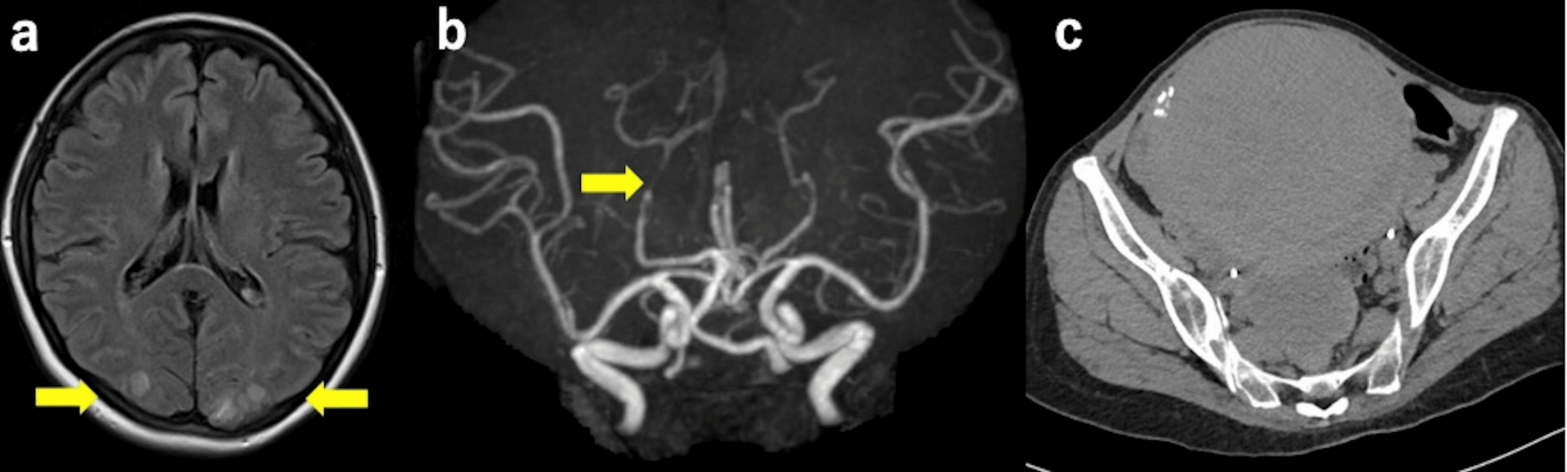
Axial FLAIR MRI (1.5 T; repetition time, 10,000 ms; echo time, 102 ms) on the day of PRES onset (a) shows bilateral hyperintensity in the occipital subcortical white matter (arrows) (a). MRA on the same day (b) demonstrates segmental narrowing of the posterior cerebral arteries consistent with vasospasm (arrow). Abdominal CT on the same day as PRES onset (c) demonstrates large uterine fibroids. FLAIR, fluid-attenuation inversion recovery; MRI, magnetic resonance imaging; PRES, posterior reversible encephalopathy syndrome; MRA, Magnetic resonance angiography; CT, computed tomography.
